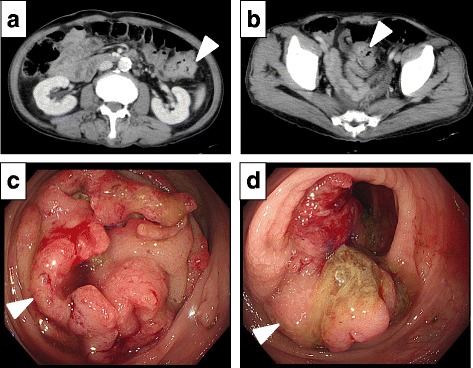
Examination images of the colon cancers before the operation. a Abdominal computed tomography revealing an enhancing mass in the sigmoid colon (*white arrow head*). b A second enhancing mass was identified in the descending colon (*white arrow head*). c Colonoscopy revealing sigmoid colon cancer (*white arrow head*). d Synchronous descending colon cancer (*white arrow head*)
